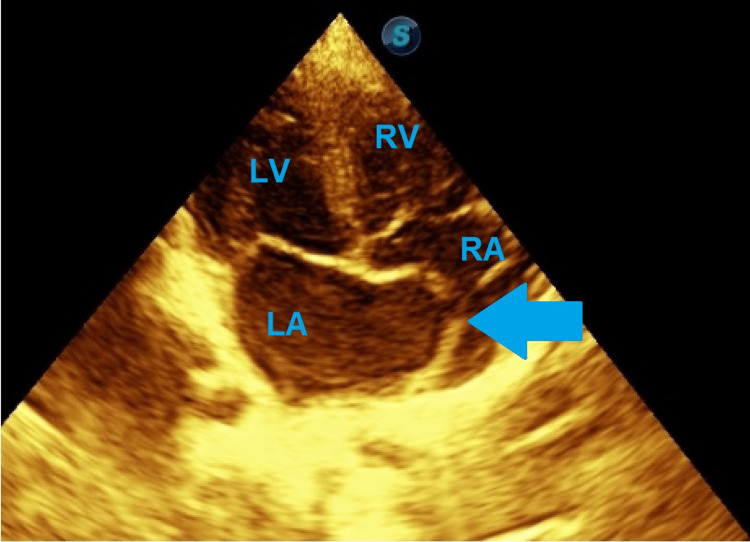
Postnatal transthoracic echocardiogram showing a large mobile aneurysm protruding into the right atrium LA: left atrium, LV: left ventricle, RA: right atrium, RV: right ventricle, Arrow: interatrial septum aneurysm
